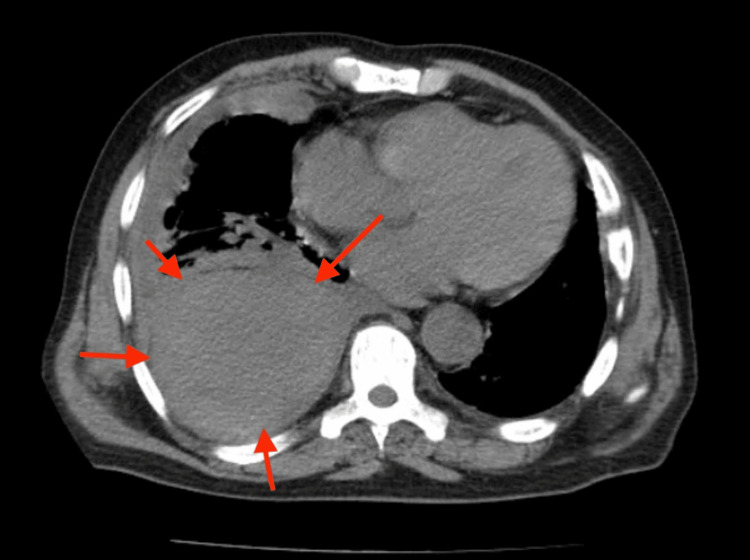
Contrast-enhanced axial CT image obtained with limited contrast opacification, showing no definite active contrast extravasation within the extrapleural hematoma. Moderate right pleural effusion is also present. The arrows indicate the extrapleural hematoma and associated pleural effusion.
